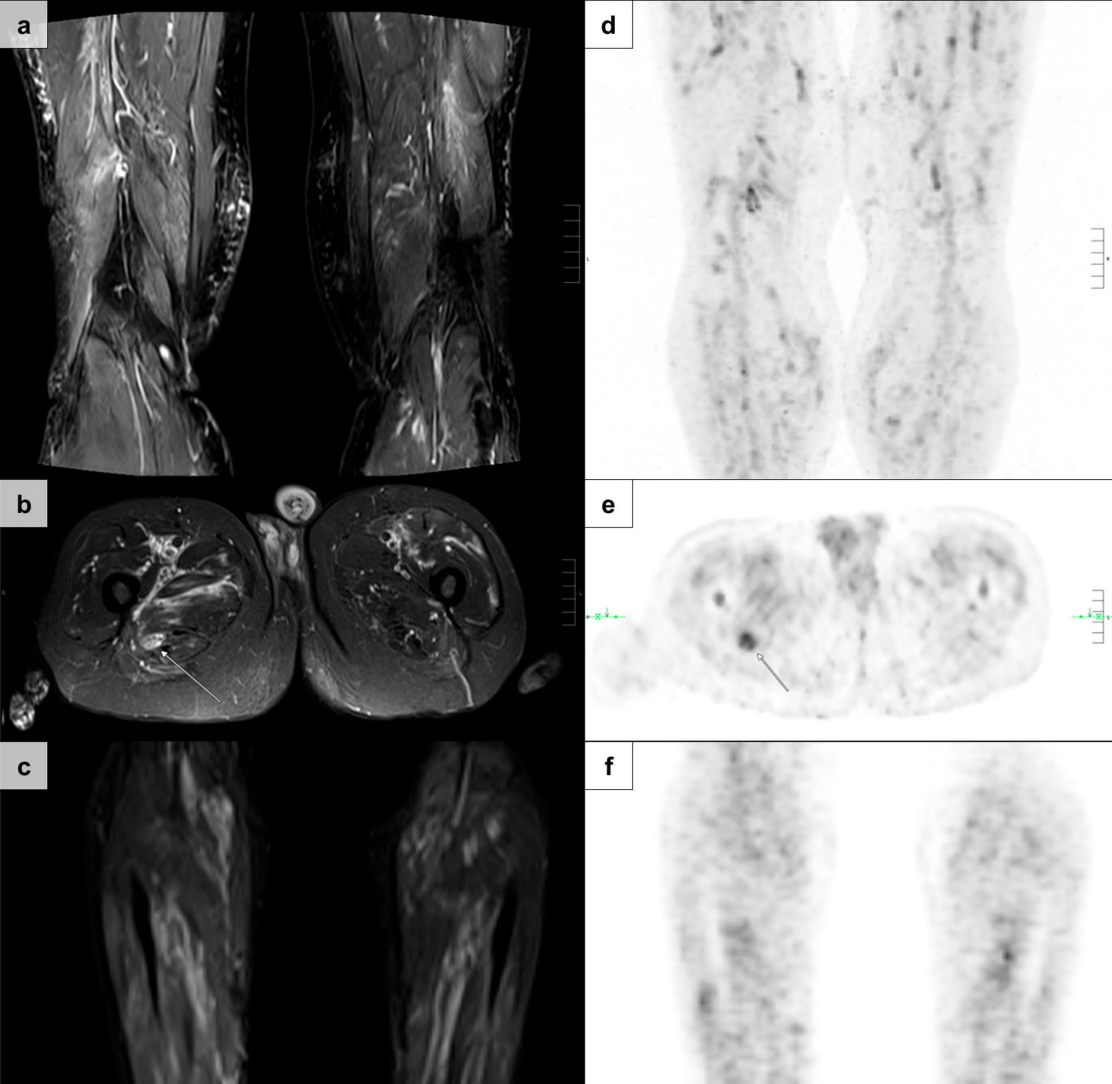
Imaging findings of vasculitic myopathy in ANCA-negative vasculitis. T2-weighted magnetic resonance imaging findings of multifocal hyperintensities pronounced in the vicinity of intramuscular arteries, consistent with edematous lesions secondary to vasculitis (a–c). Corresponding whole body [^18^F] fluorodeoxyglucose positron emission tomography imaging demonstrates increased radiotracer uptake in peri- and intramuscular arteries of the lower extremities—referred to as the ‘dirty muscle sign’ (d–f)
